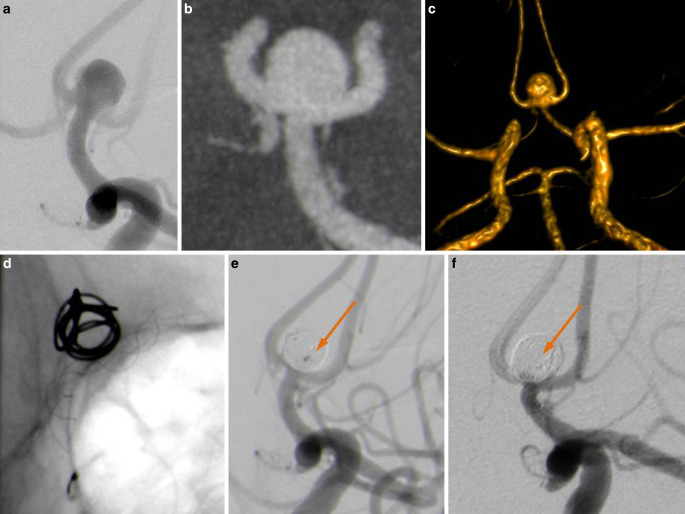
Wide-neck Acom aneurysm involving both A2 branches (a, b). FD-CT with iv contrast (c) shows dominant feeding of both A2 branches from the left side. Note the two helical marker strands (d) indicating complete opening of the stent. *Arrow* in e marks residual central inflow (MRRC3a) by the end of the procedure which had disappeared at follow-up (f). a, b and d–f show DSA images
